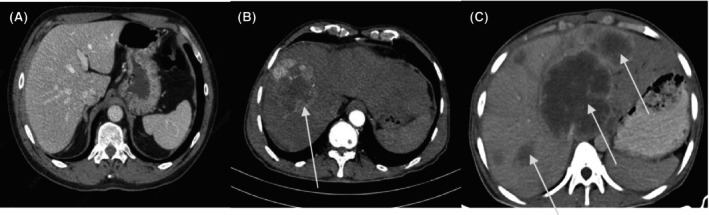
(A) Normal liver on CT scan. (B) Hepatocellular carcinoma. (C) Multiple liver lesions—Metastasis (arrows indicating the malignant lesion).
